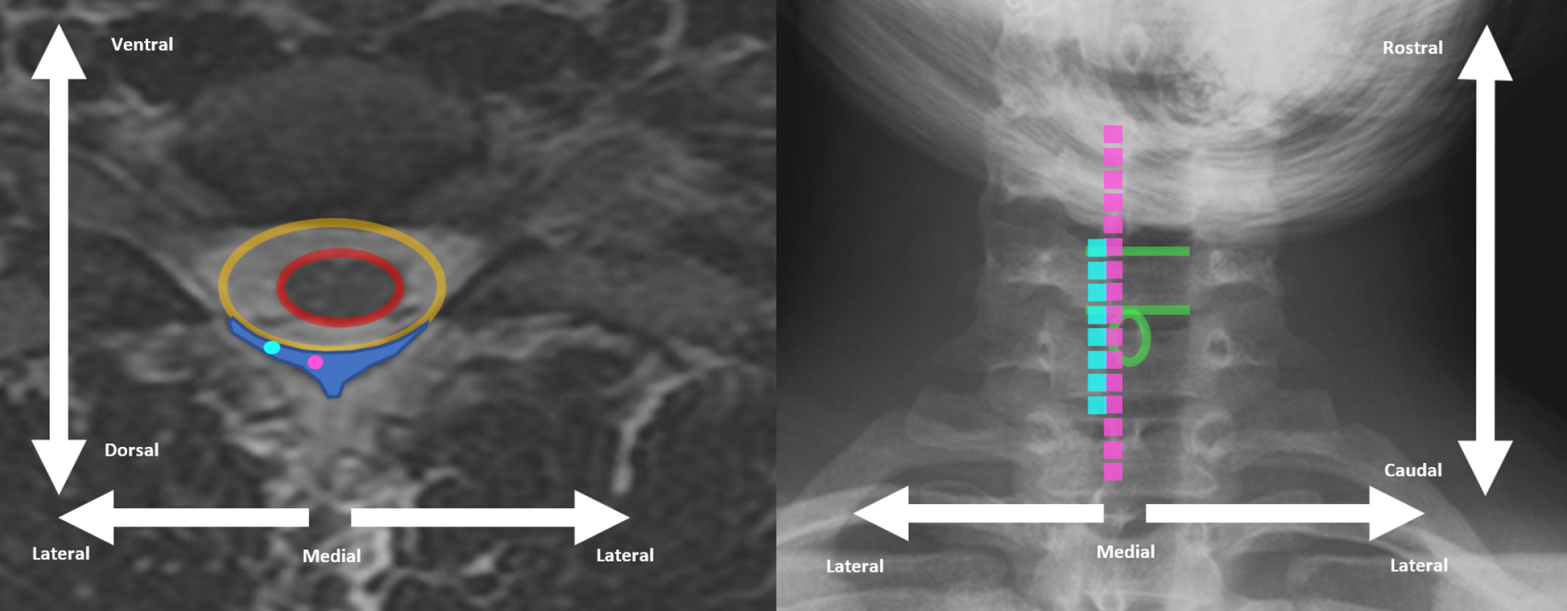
Example cervical spine MRI axial view (left) and cervical spine x-ray (right) demonstrating differences between traditional dorsal column SCS placement (pink) and more lateral placement (light blue). Spinal cord is encircled in red, dural space is encircled in orange, and epidural space is indicated by dark blue shading on left. Superior and inferior end-plate of example vertebral body indicated with green line on right. Spinous process of same vertebral level encircles in green circle on right.
